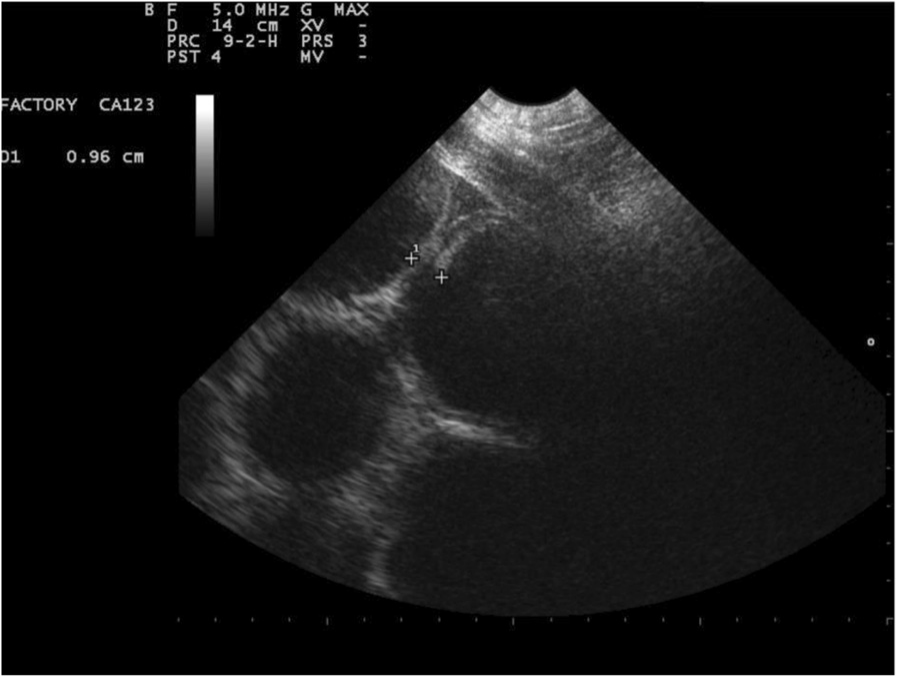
Abdominal ultrasound of the gelding with tympanic colic as a result of anaphylactic reaction to sodium ceftriaxone. Image obtained by ventral-abdominal positioning of the probe, caudal to xiphoid. Note the thickening of intestinal wall, distention, atony and *ileus* in segments of the small intestine. Diagnostic Imaging Sector - FCAV - UNESP - Jaboticabal
